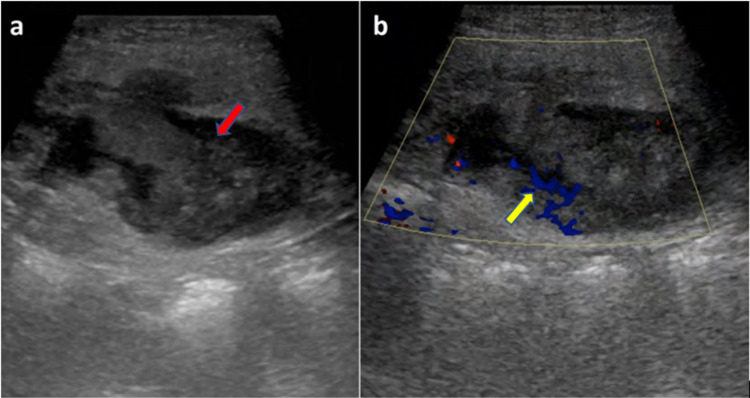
Ultrasound of abdomen showing a) a mixed solid and cystic lesion with irregular margins in the deep anterior abdominal wall in epigastrium with mild edema of adjoining soft tissue (Red arrow) and b) minimal peripheral vascularity (Yellow arrow).
